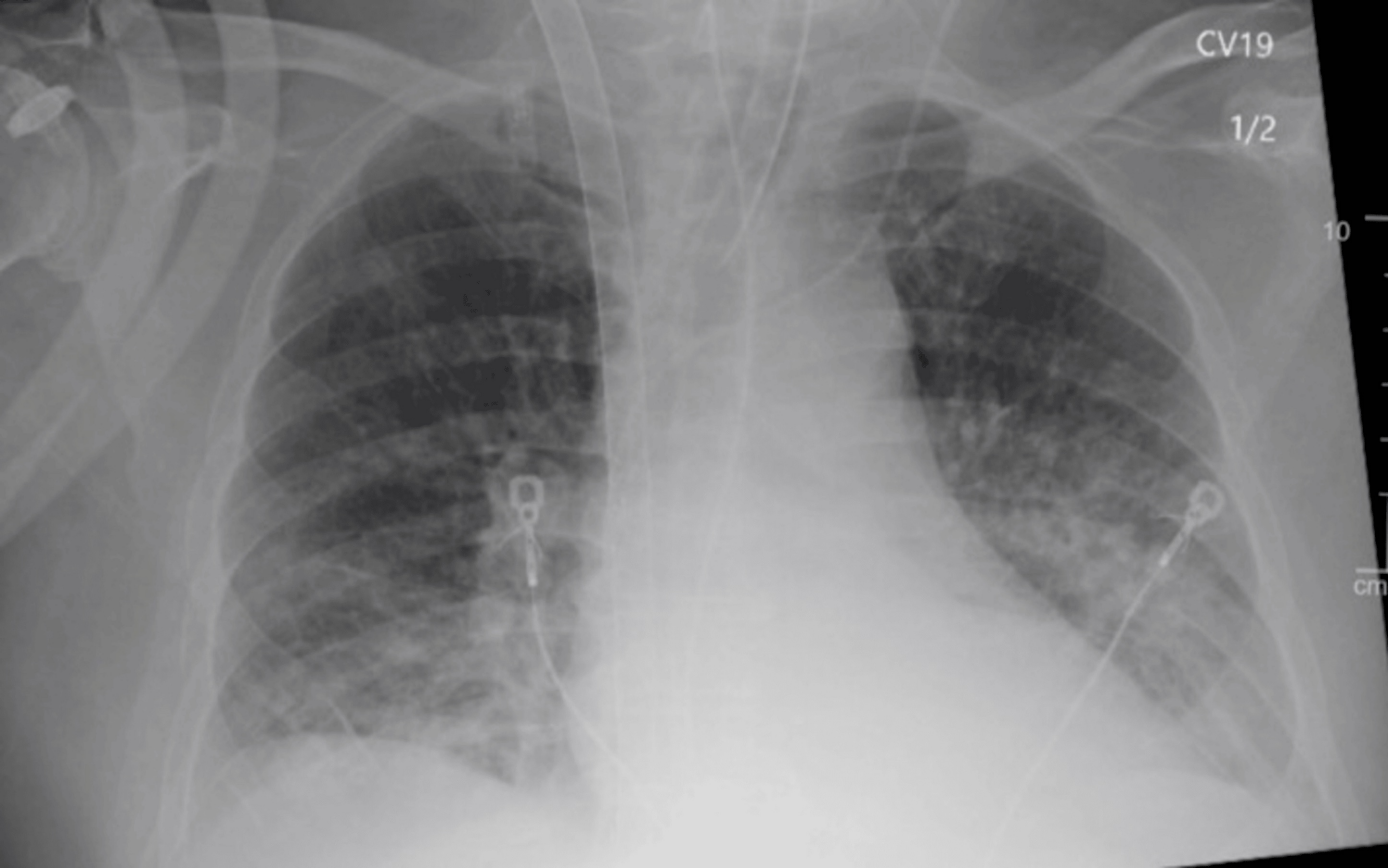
Chest X-ray just prior to V-V ECMO decannulation. Improved aeration is seen bilaterally.
